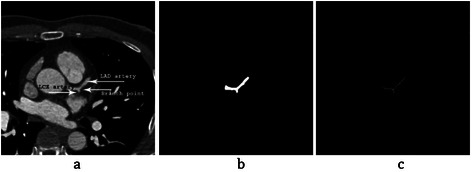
LAD and LCX seed point detection. (a) A CTA slice includes LM artery. (b) Segmented region currently saved in matrix *H*. (c) Thinning by means of morphological operation for bifurcation detection.
